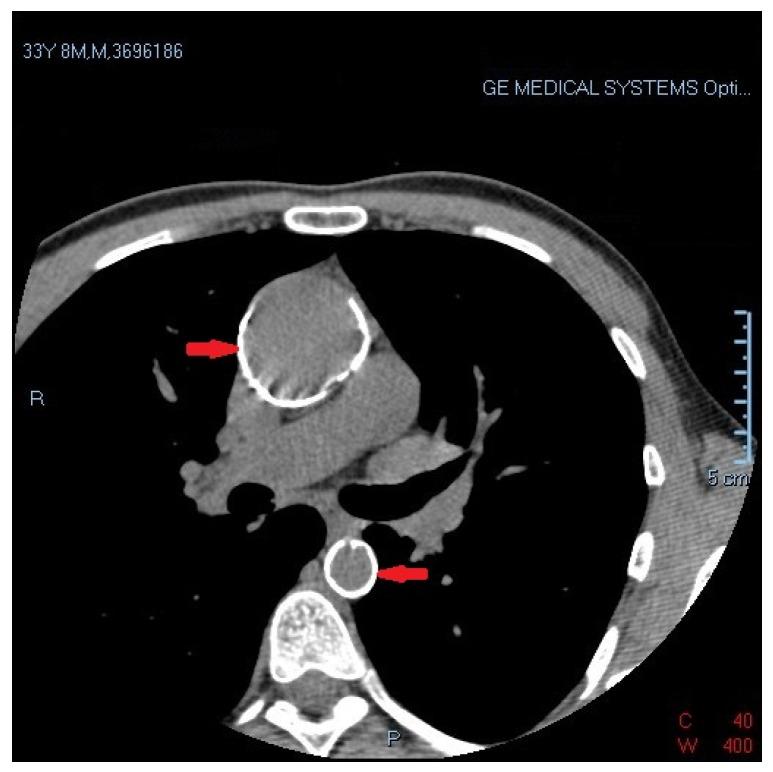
Computed tomography (CT) confirmed severe ascending and descending aortic calcification compatible with porcelain aorta.
